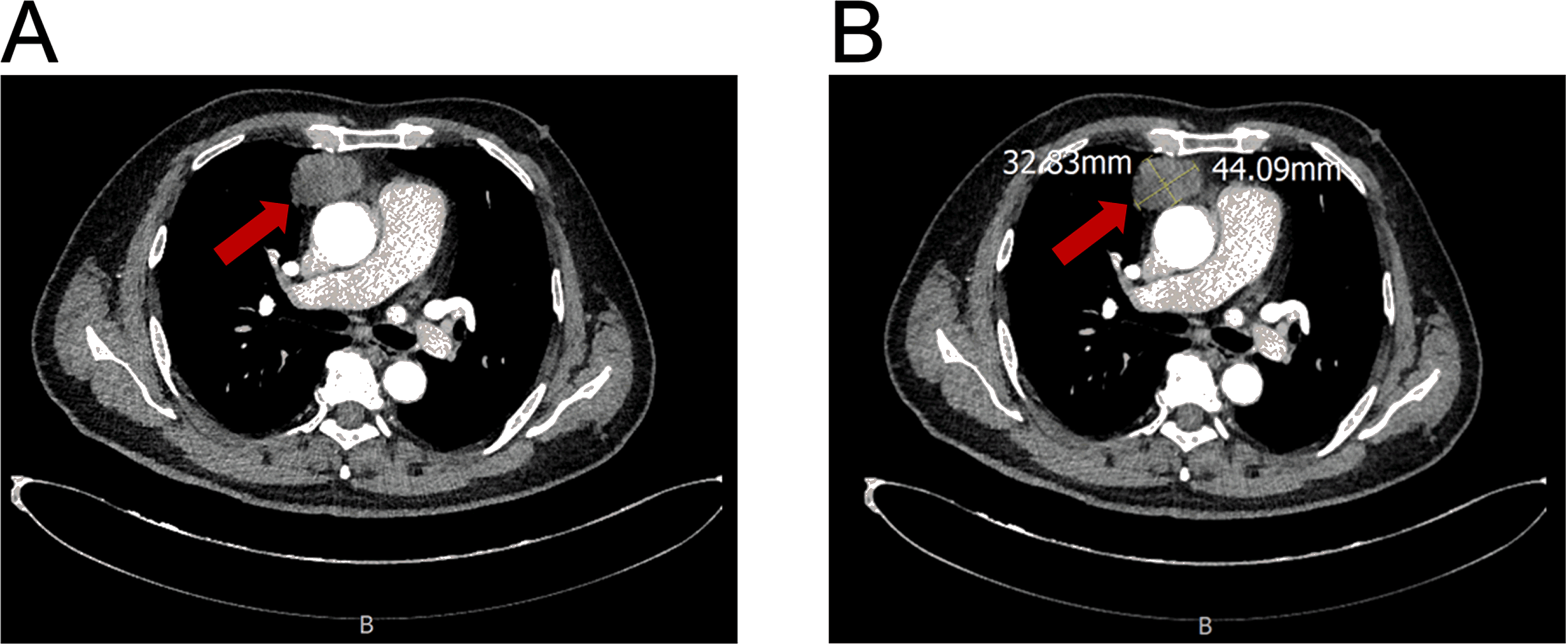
Contrast-enhanced CT of an anterior mediastinal mass. (A) Axial image showing the mass (arrow). (B) Image demonstrating tumor measurement (44.09 mm x 32.63 mm).
